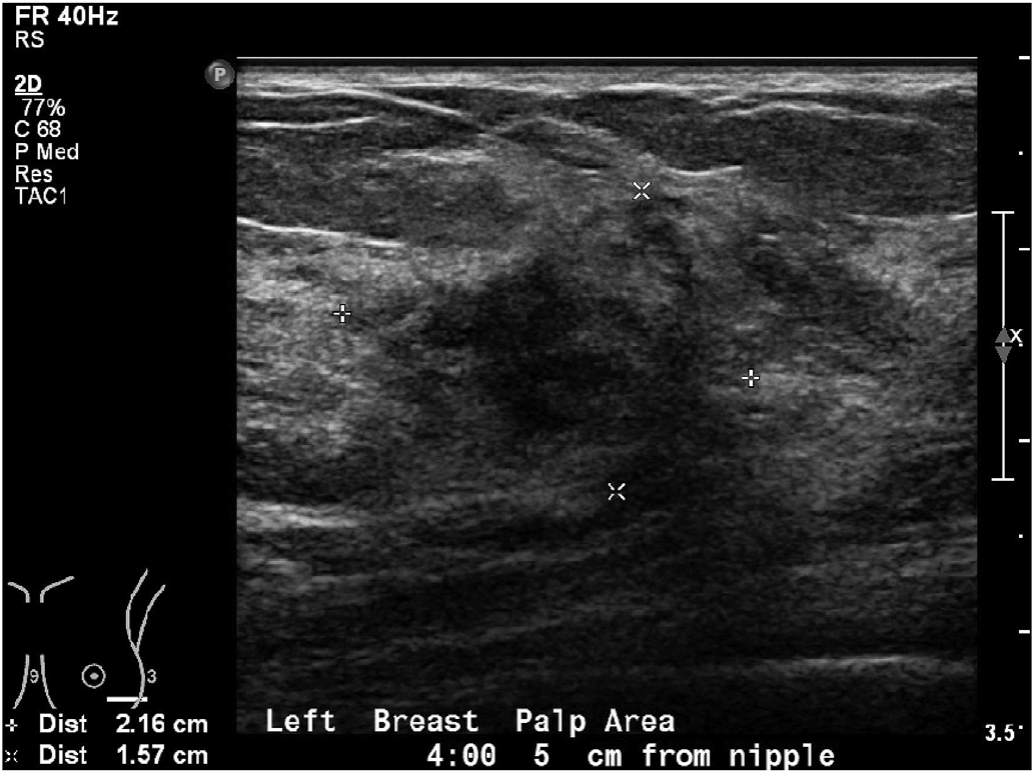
Ultrasound left breast the region of palpable abnormality shows an area of heterogeneous hypoechoic tissue at approximate 4:00 o'clock, 5 cm from the nipple measuring up to 2.2 × 1.6 cm.
